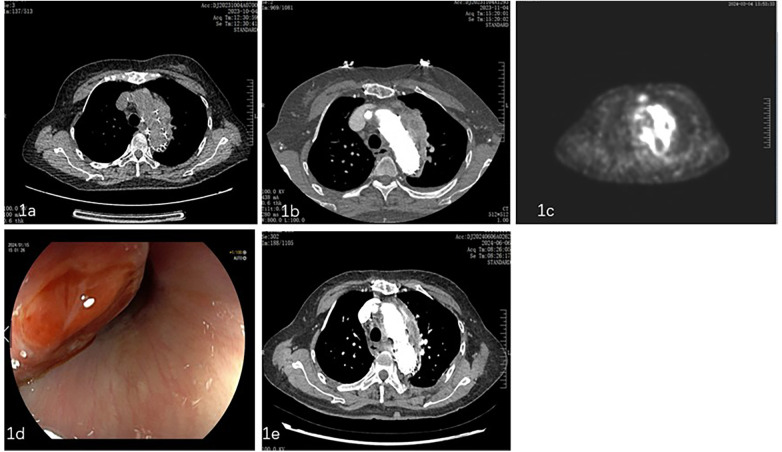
(a,b) chief complaint of chest and back pain with dysphagia, CT suggests mediastinal abscess. (c) 18F-FDG PET-CT suggests a lobulated soft tissue mass in the left upper mediastinum with increased metabolism, and localized increased metabolism in the middle esophagus, suggesting a possible infectious lesion. (d) Gastroscopy suggests external compression of the upper esophagus. (e) Postoperative CT scan showed a significant reduction in mediastinal foci.
